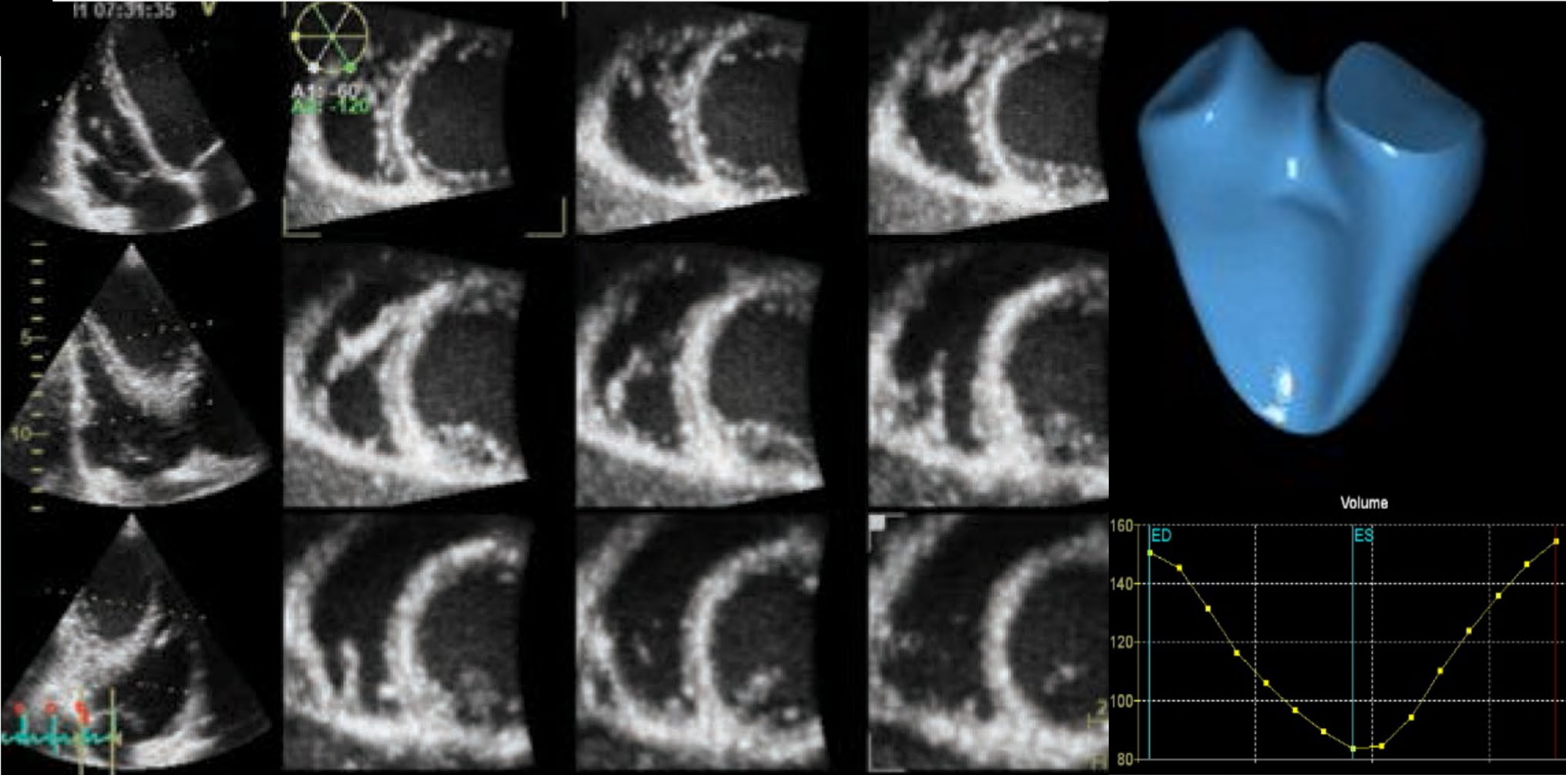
Three-dimensional echocardiographic measurements of the right ventricular volumes and ejection fraction. A 72-year-old man presented with severe secondary TR. Two-dimensional echocardiography showed a non-dilated right ventricle (basal diameter 39 mm) and a preserved tricuspid annular plane systolic excursion (18 mm). In contrast, three-dimensional echocardiography shows a mildly dilated right ventricle (end-diastolic volume 91 ml/m.^2^) with an impaired right ventricular ejection fraction (42%)
